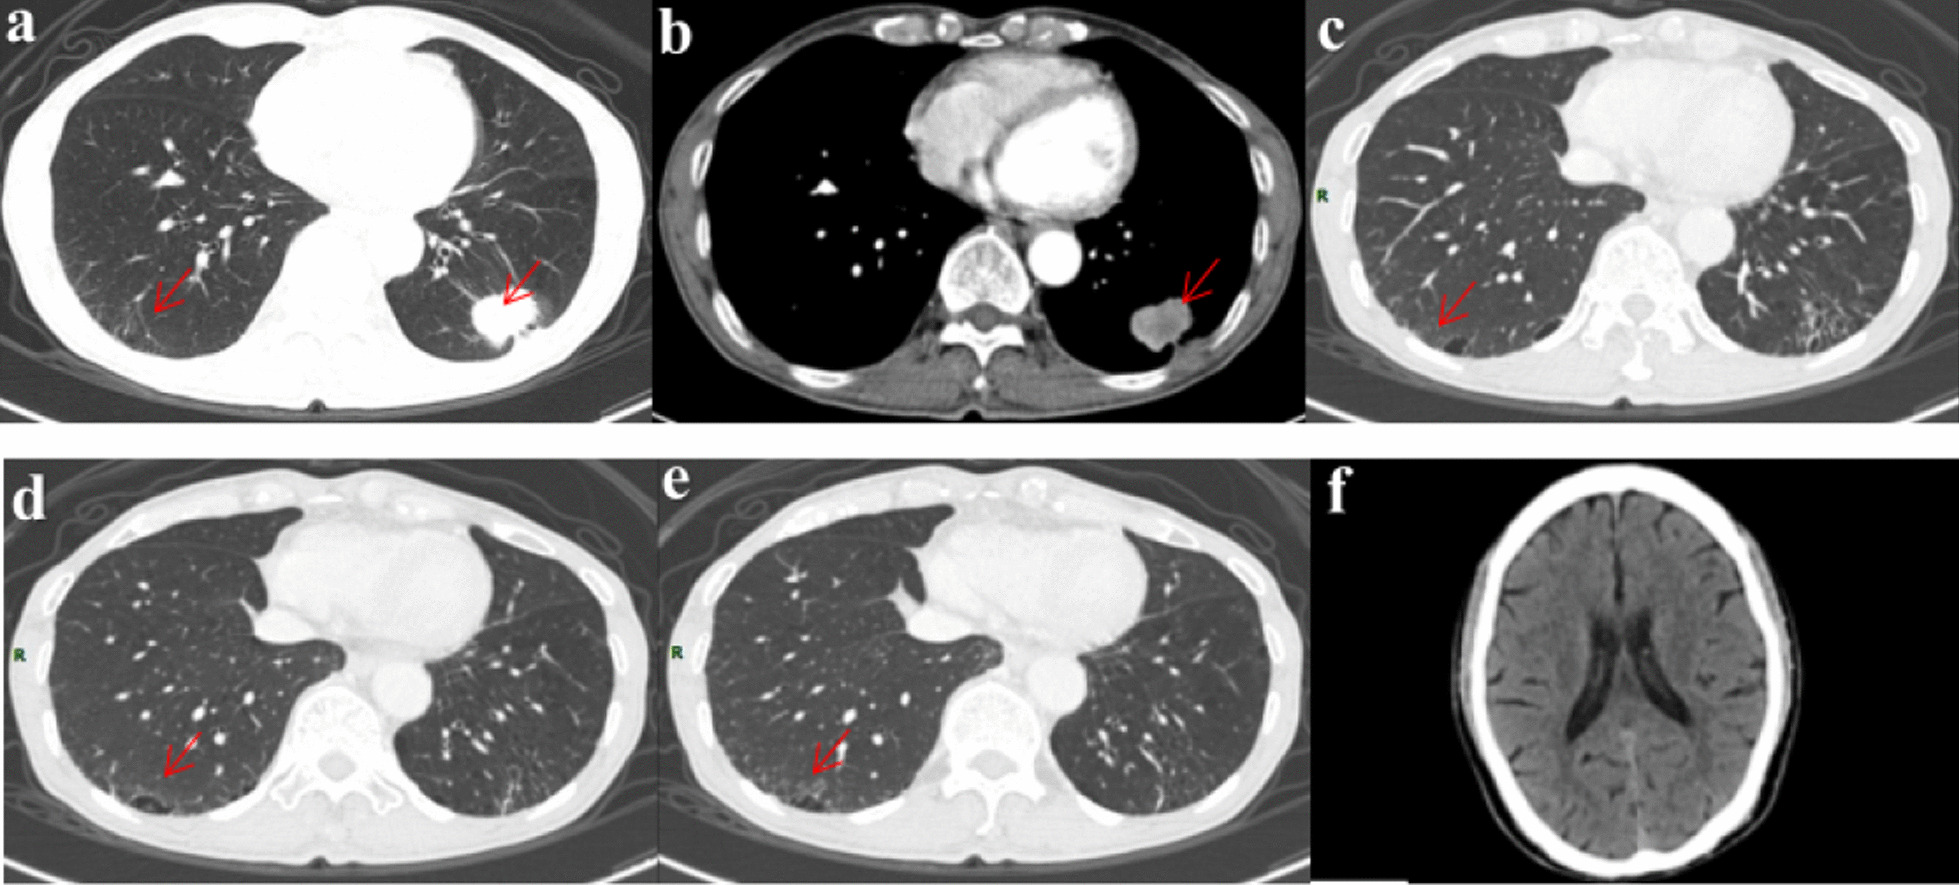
Preoperative CT of the chest and head. a–b A nodular shadow of 22 mm × 31 mm located in the lower lobe of the left lung with irregularly lobulated shape, spiculation and pleural invasion. c–e Some reticular opacities and a few ground-glass opacities can be seen in the right lower lung. f No metastasis was found in head CT scan
